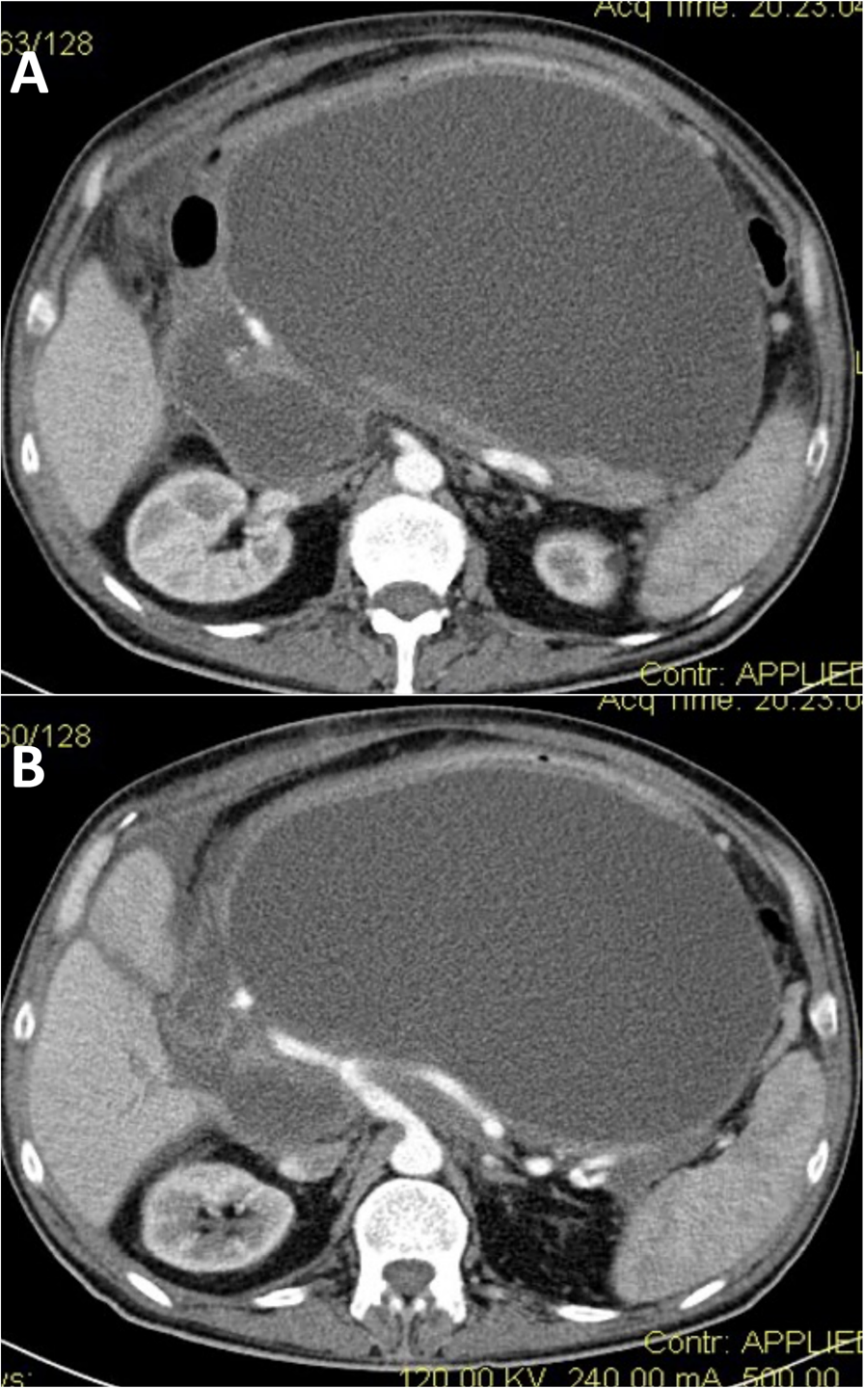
Case 2: Preoperative CT scan. a, b CT scan showing a heterogeneous and sepimented 23 × 15-cm low-density lesion suggestive for infected pancreatic necrosis
